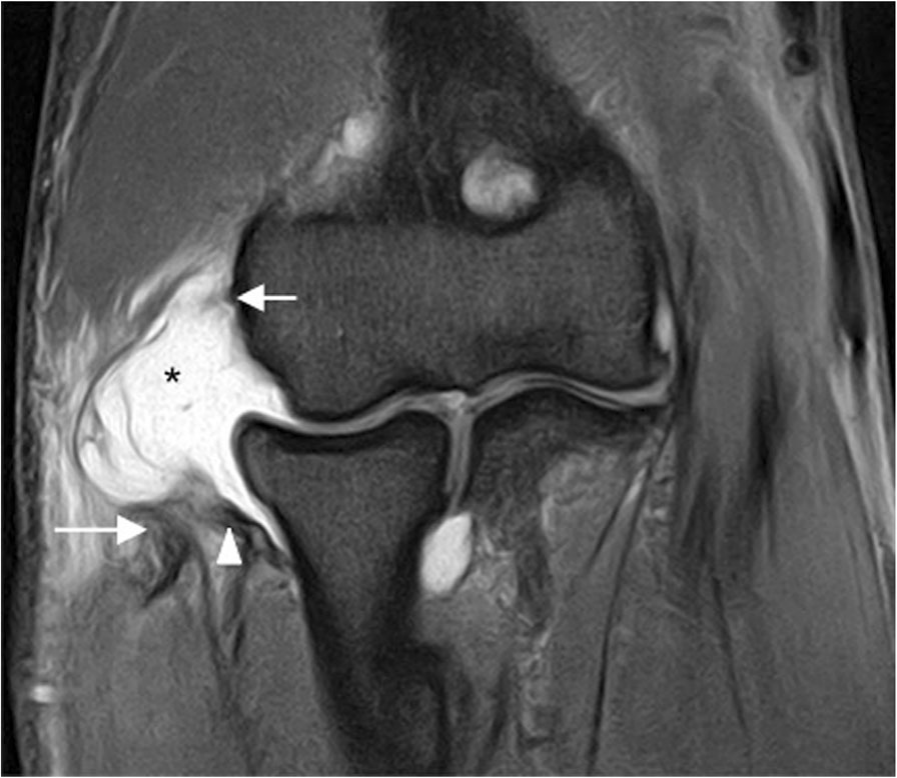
Posterolateral rotatory instability, stage 2. Coronal FS PD-weighted MRI showing a complete detachment of the proximal lateral ulnar collateral ligament, the proximal radial collateral ligament proper, and the common extensor tendon (white short arrow), with retraction of the lateral ulnar collateral ligament (white arrowhead) and common extensor tendon (long white arrow). Note the extravasation of the joint fluid through the tear (black asterisk)
